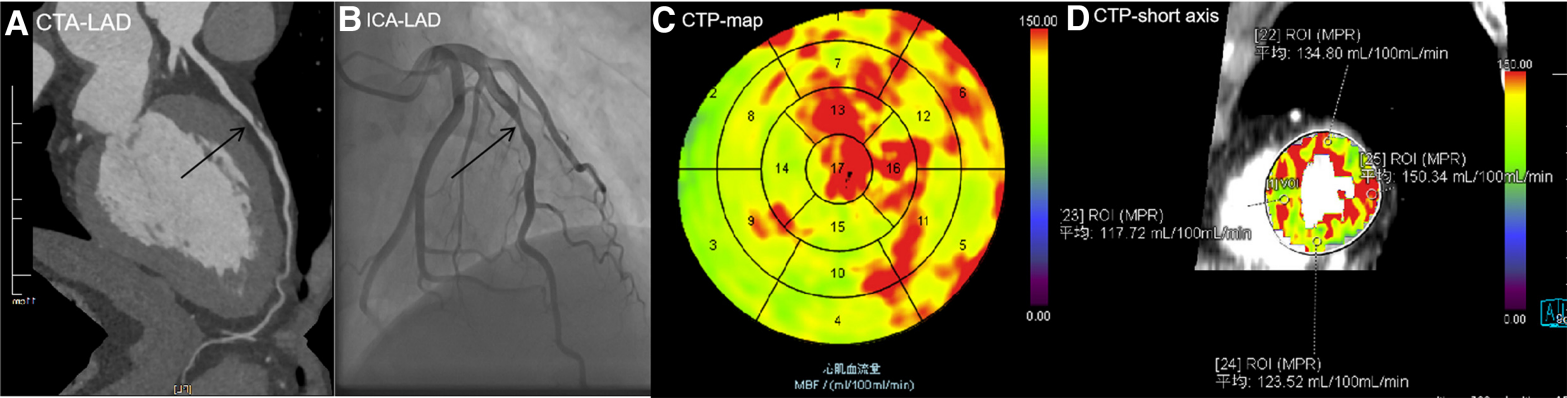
A 56-year-old man with type 2 diabetes for 1 year presented with chest pain. (A) CCTA image showing atherosclerotic plaques in the middle of the LAD with severe stenosis of 80%, indicating MI. (B) ICA showing 75% stenosis in the middle of the LAD, with an FFR value of 0.85. No revascularization therapy was performed. (C) CTP-MBF map image showing that the LAD territory (segment 14) was relatively lower than other areas. The MBF-global value was 131.15 ml/100 ml/min. (D) MBF short-axis image showing that all MBFa values were higher than the cutoff value (117.71 ml/100 ml/min), the lowest MBF-ratio value in segment 14 was 0.783 (117.72/150.34), and there was no evidence of MI. In this patient, moderate stenosis of the coronary artery did not cause MI, and MBFa and MBF-ratio values were consistent with FFR. LAD, left anterior descending artery; CTP, computed tomography perfusion.
